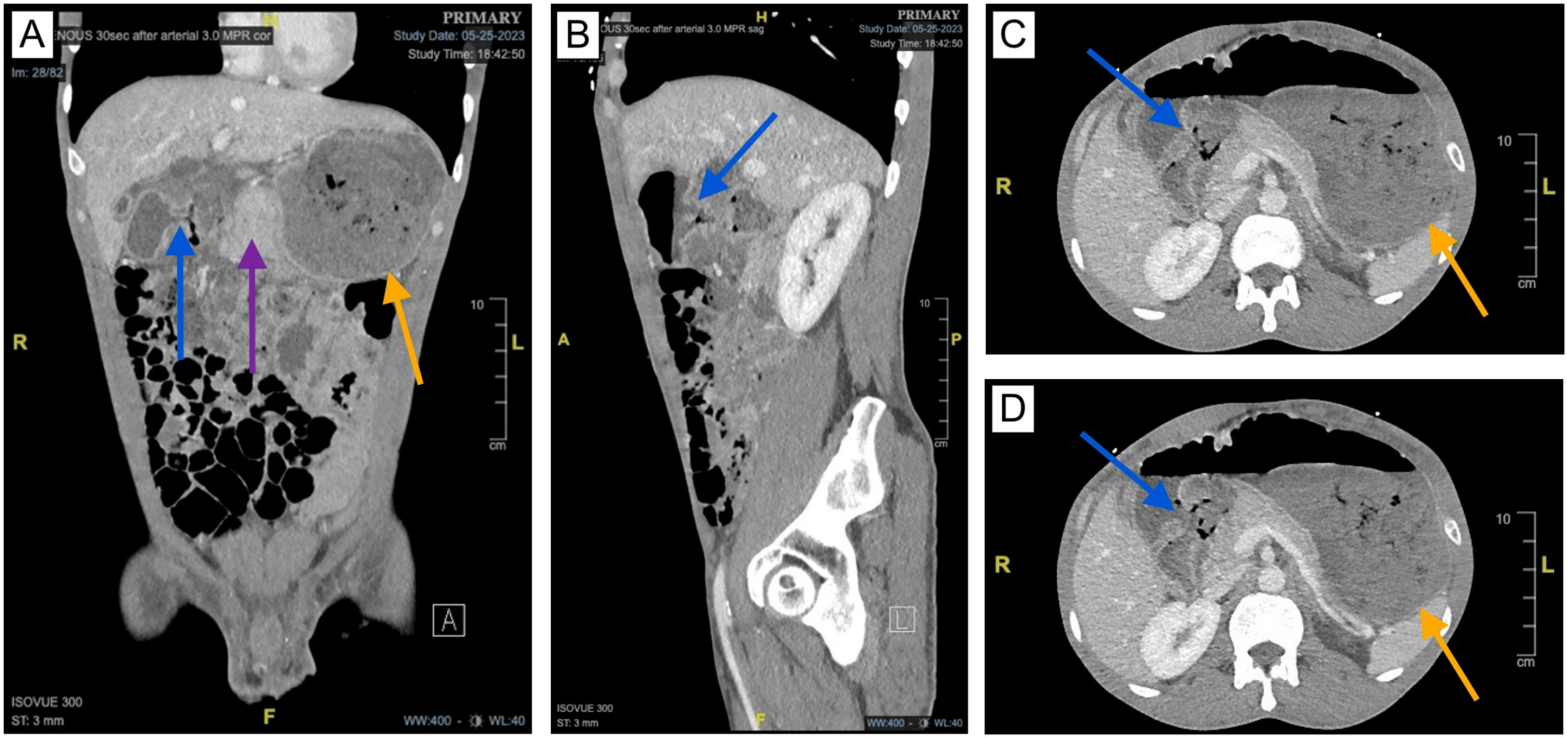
A CT without contrast demonstrates features of peptic ulcer disease of the duodenum. (A/B) Coronal and sagittal views show severe edema of the gastric pylorus and duodenum (purple arrow) as well as a possible contained perforation of the duodenal wall (blue arrows). (C/D) Axial views show the possible duodenal ulcer perforation that is walled off (blue arrows). The severe edema may be causing gastric outlet obstruction, which can be appreciated by the severely distended stomach with retained gastric contents (orange arrows).
